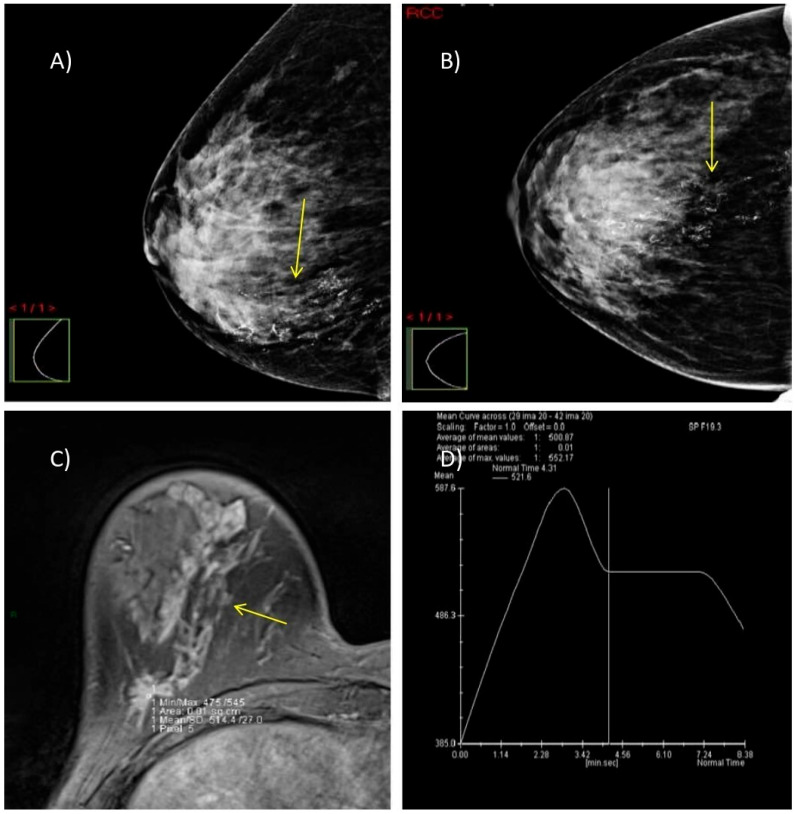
A case of a 47-year-old woman with dense breasts (ACR category C) and HPE-verified multifocal invasive ductal carcinoma, grade 2 in the right breast. (A,B) DM in MLO and CC projections shows highly suspicious microcalcifications with segmental distribution at the junction of the lower breast quadrants (yellow arrow). (C) Axial 3D T1-weighted FLASH fat-suppressed MR image demonstrates an extensive post-contrast hyperintense mass lesion extending from the nipple to the prepectoral space (yellow arrow). (D) MRI dynamic contrast enhancement curve type III (“wash out” curve).
